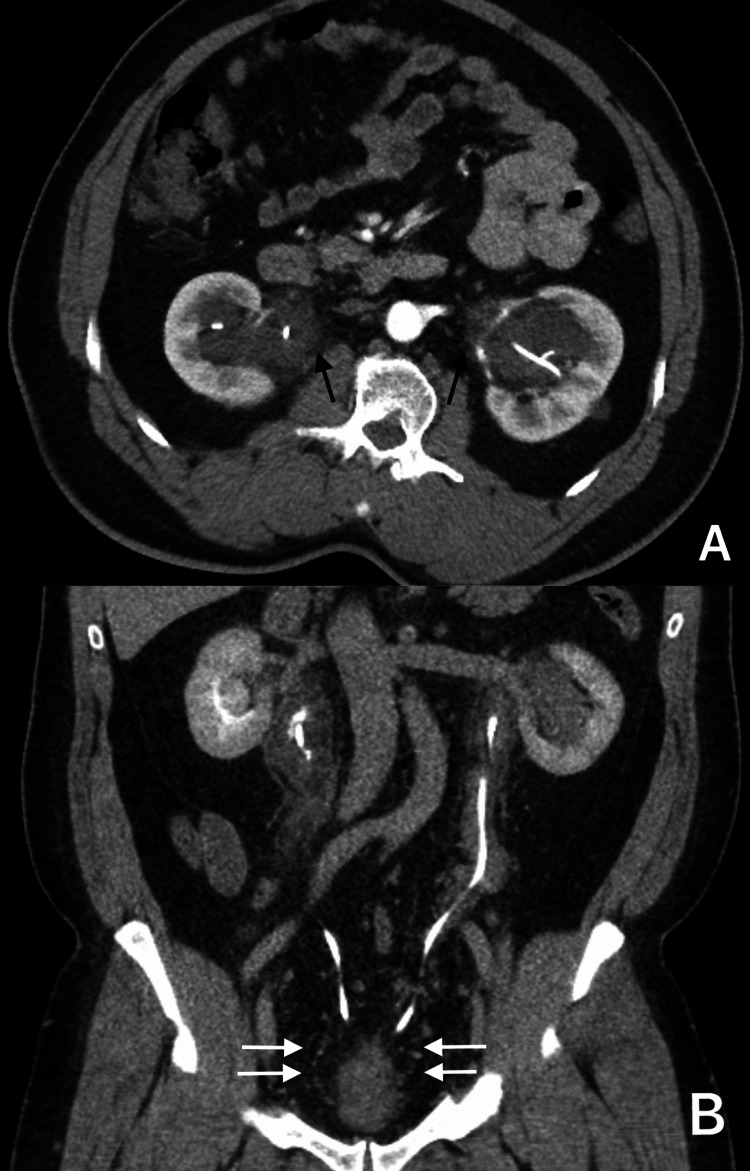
Pre-operative CT (axial and coronal view) (A) axial view, (B) coronal view. Pelvic lipomatosis (white arrows) causing moderate bilateral hydroureteronephrosis (black arrows). The finding of bladder wall thickening with peri-vesical and periureteric stranding.
